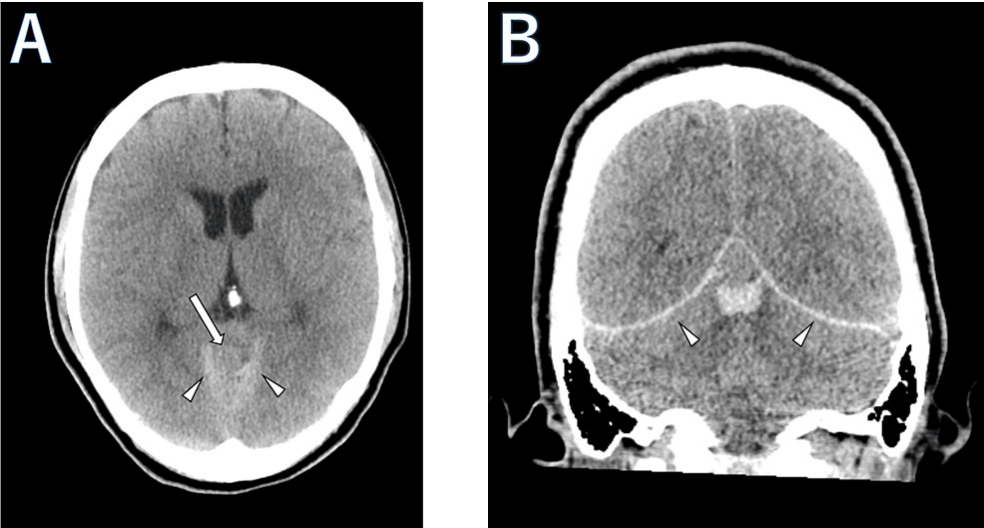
Preoperative non-enhanced computed tomography (CT). (A) Axial and (B) coronal images show a hemorrhagic mass (arrow) measuring 1.6 cm × 1.4 cm × 1.2 cm under the cerebellar tentorium with the isodensity as cortical gray matter, with bleeding observed below the mass. No calcification was present. An acute subdural hematoma extending along the cerebellar tentorium (arrowhead) was demonstrated.
